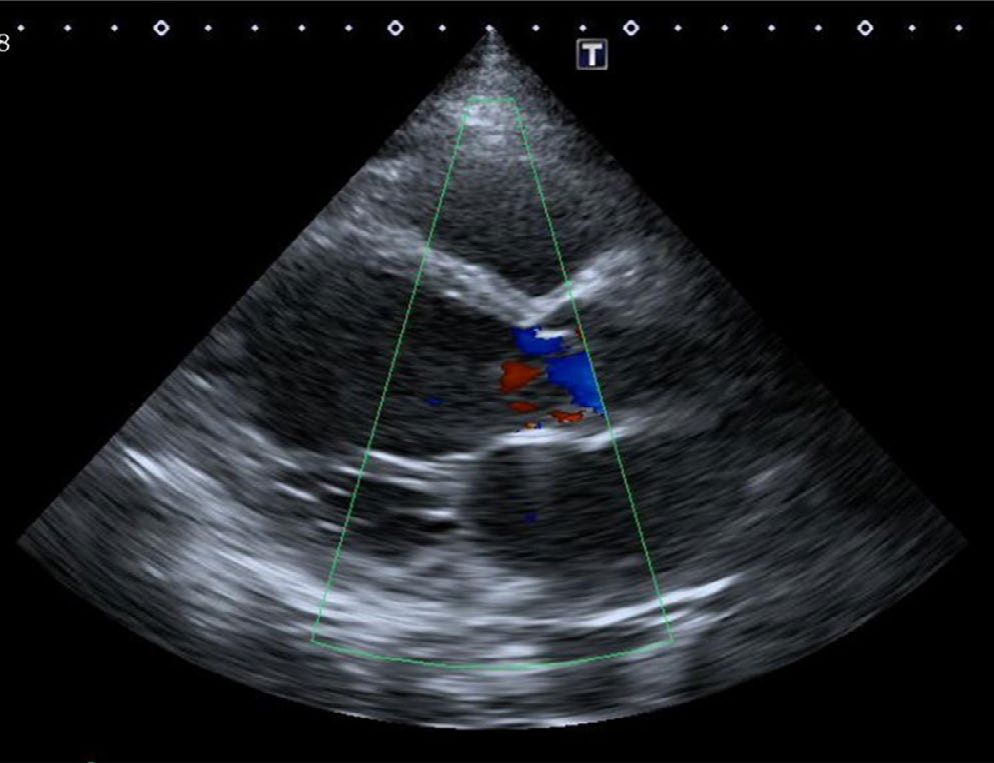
Long‐axis view on echocardiography at the time of discharge. Echocardiography showed diffuse severe hypokinesis, but LVEF and mitral regurgitation had improved compared to the time of admission
